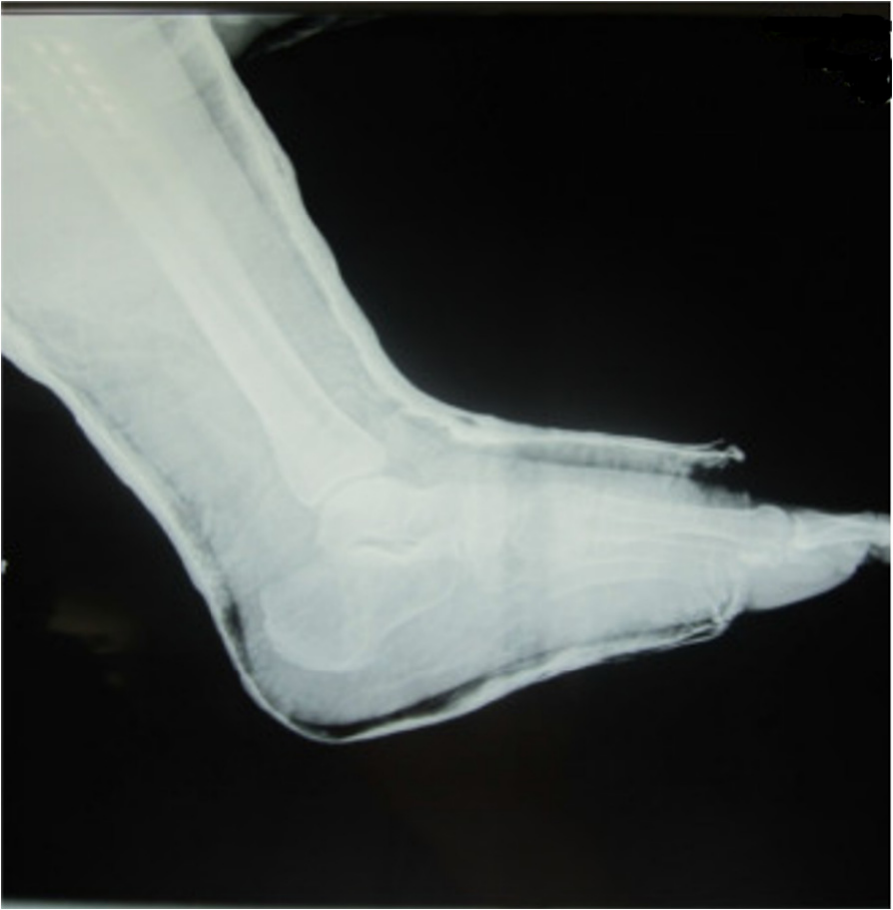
Control lateral radiographs after reduction.
